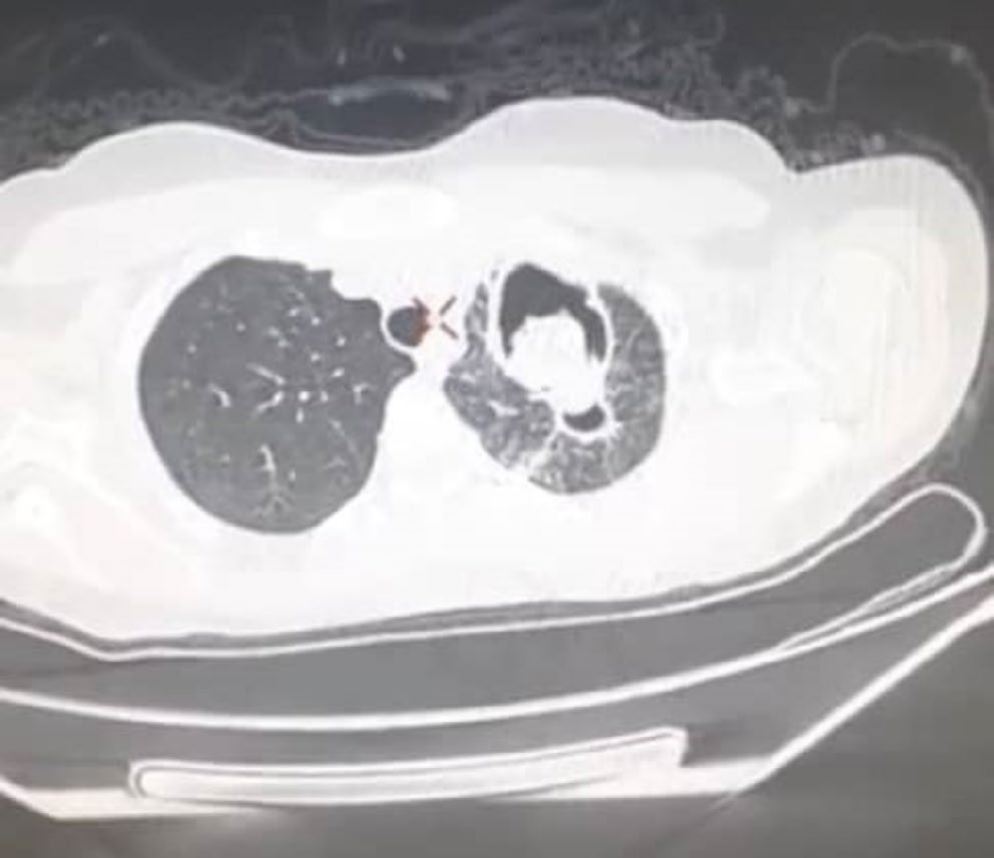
Axial chest CT scan (lung window) confirming the presence of an air‐fluid level within the left upper lobe cavity, suggestive of cyst rupture or secondary infection.
